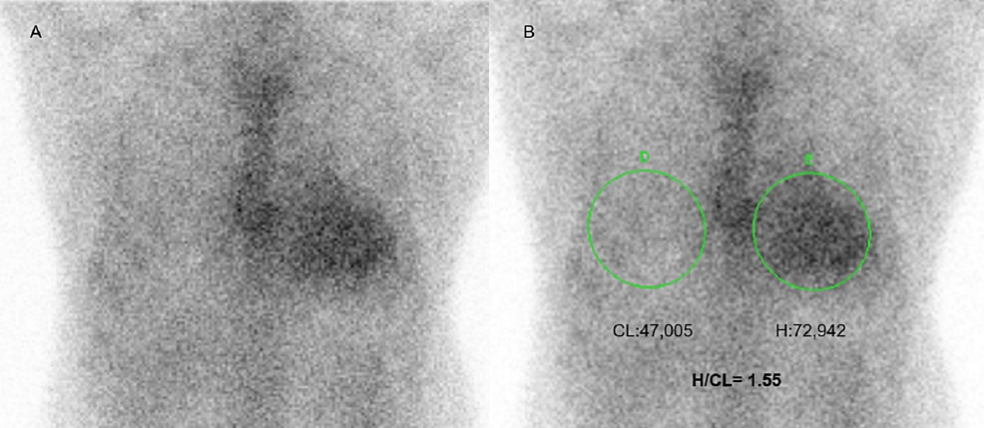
Technetium-99m pyrophosphate scintigraphy- planar imaging Pyrophosphate scintigraphy in anterior projection shows myocardial uptake more than rib (A). On quantitative analysis, H/CL is 1.55 (B).
